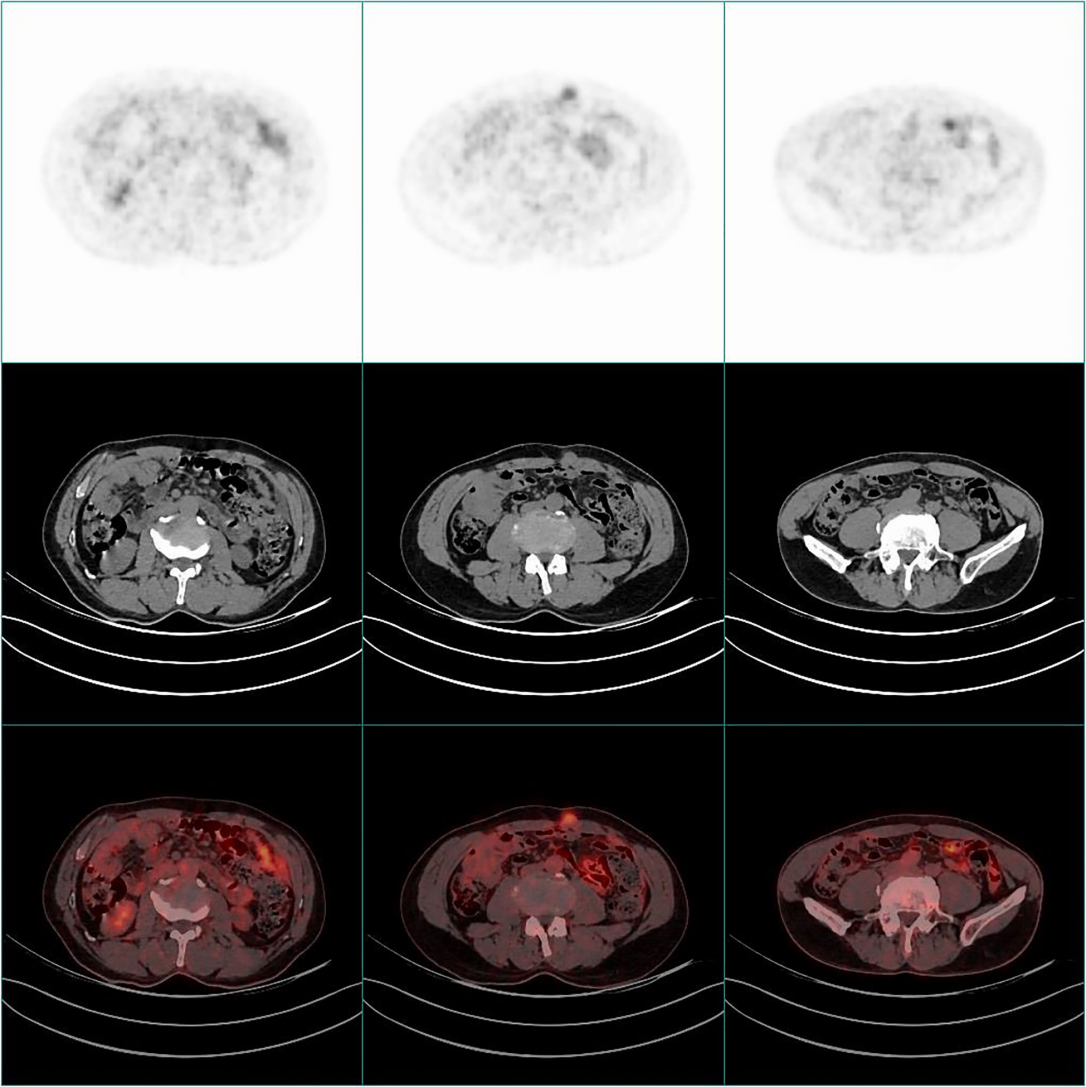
PET/CT scan of a 69 year old male patient with soft tissue metastasis in the anterior abdominal wall. PET images and fusion PET/CT and CT axial images showed a FDG positive nodule (SUVmax=3.4) near the anterior abdominal wall navel, accompanied by diffuse thickening of the peritoneum and increased FDG uptake. Pathological indication: malignant mesothelioma.
